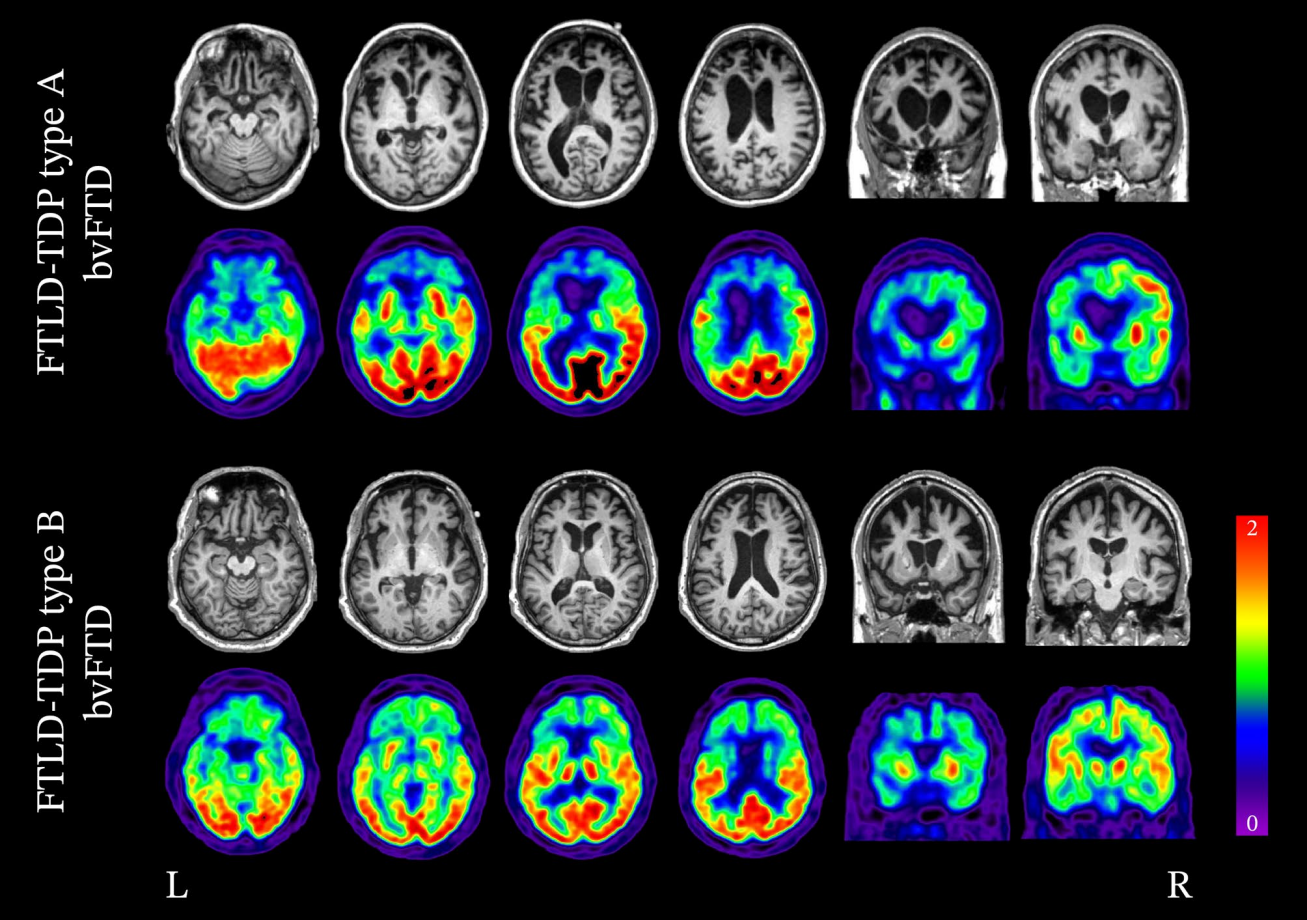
Neuroimaging of bvFTD associated with FTLD-TDP type A and B (top row: structural MRI; bottom row: FDG-PET). The variability in patterns of degeneration attributed to FTLD-TDP neuropathology is readily seen in these two cases of bvFTD. In the case of bvFTD associated with FTLD-TDP type A, degeneration and hypometabolism of the bilateral frontal lobes are present; however, the left is significantly more impacted than the right and extends to the left parietal lobe. In bvFTD associated with FTLD-TDP type B, significant degeneration of the bilateral frontal lobes is seen; however, in contrast to type A, the parietal lobes are less affected and atrophy is relatively symmetrical
